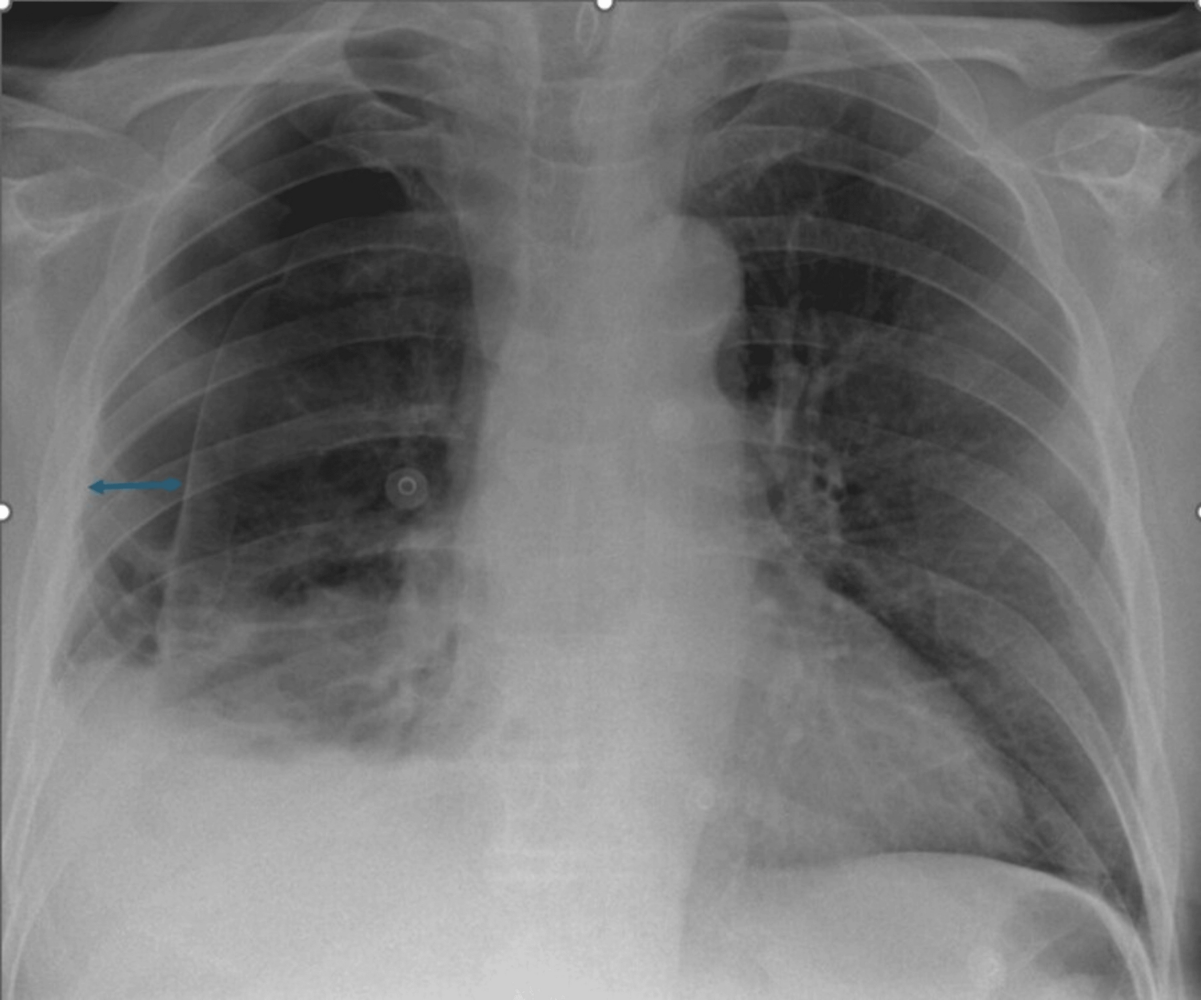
Chest X-ray showing right-sided pneumothorax at presentation
